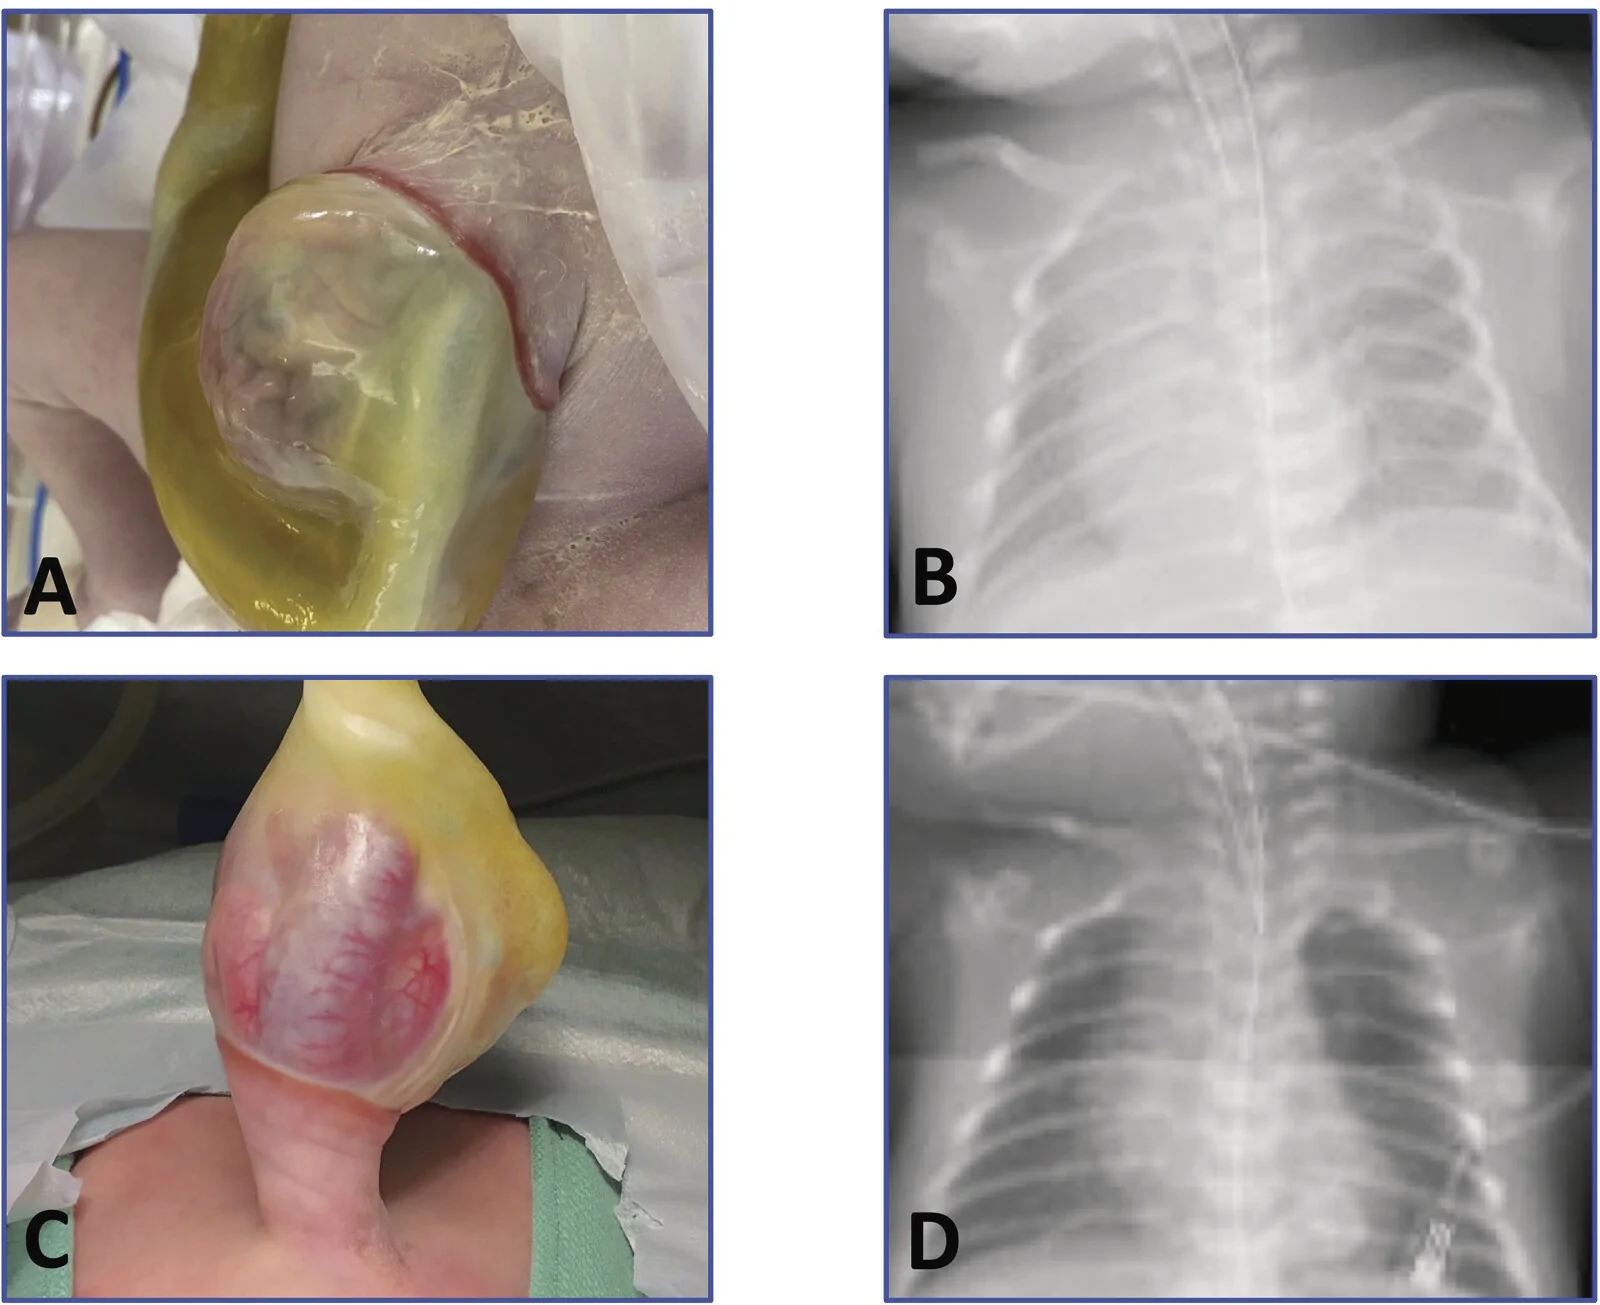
Intraoperative findings and postoperative chest radiograph in Cases 1 and 2. (
